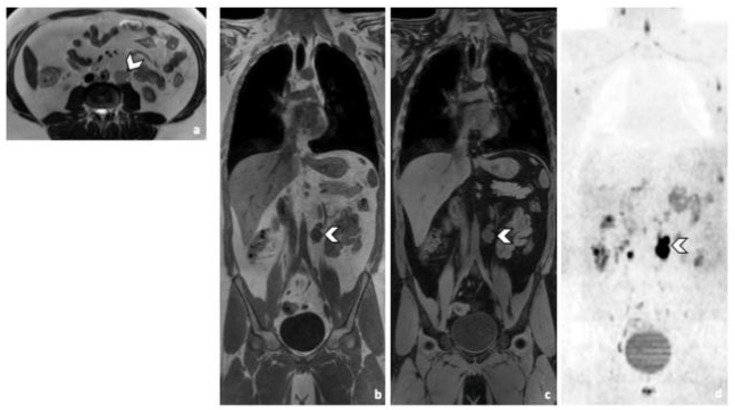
Comparison of WB sequences in a 30-year-old patient with seminoma. Axial T2-weighted (a) and coronal reformatted 3D T1 GRE In Phase (b), 3D T1 GRE Fat Sat (c) and high b-value DWI (inverted greyscale, b = 1000 s/mm^2^) MR images of the whole body (d). Detection of an enlarged pathological para-aortic lymph node (arrowhead) demonstrating an intermediate signal intensity in T2 (a), a hypointense homogenous signal in T1 (b,c) and a restricted Diffusion (d). In this case, each sequence clearly depicted the retroperitoneal lymph node. Abbreviations: 3D: Three Dimensional, T1 GRE: T1 Gradient Echo, DWI: Diffusion Weighted Imaging.
